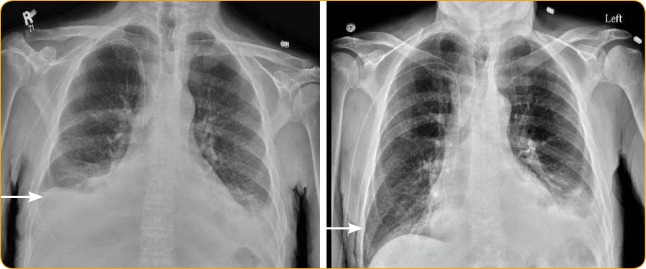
Chest x-rays show right-sided pleural effusion (left) and the same patient 1 day after drainage (right).
